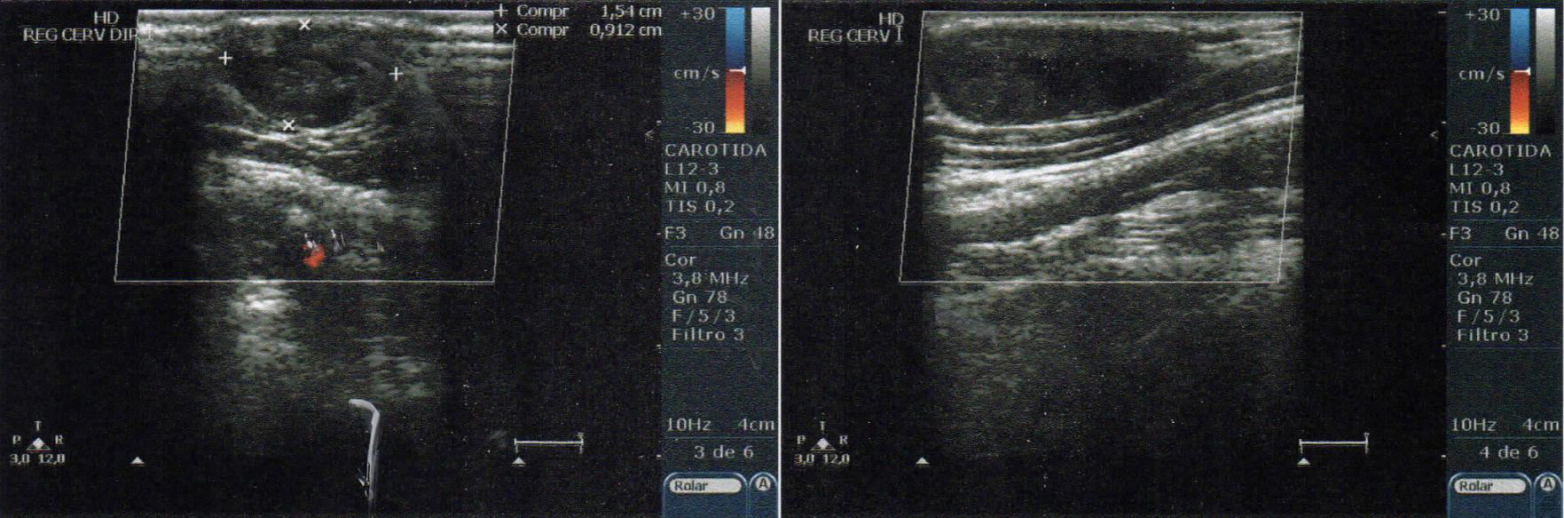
Doppler ultrasonography showing hypoechoic mass and thrombosed external jugular vein, with no flow.
